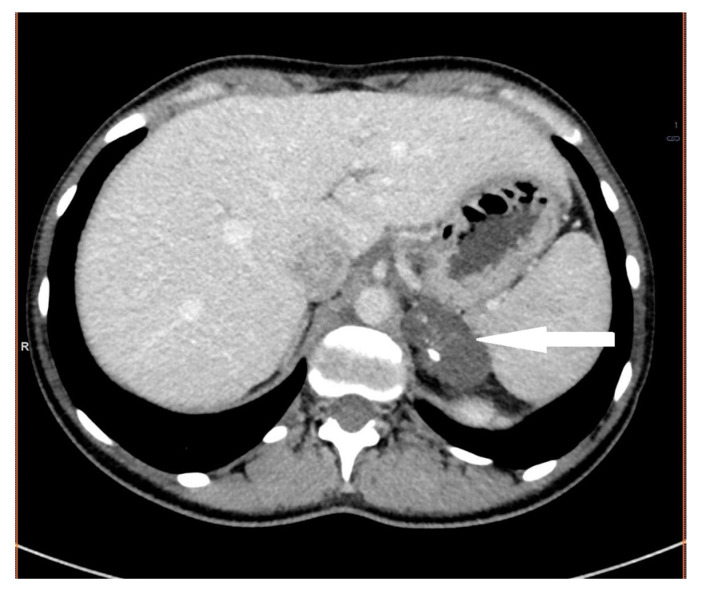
Endothelial cyst. Abdomen CT, transverse cross-section.
